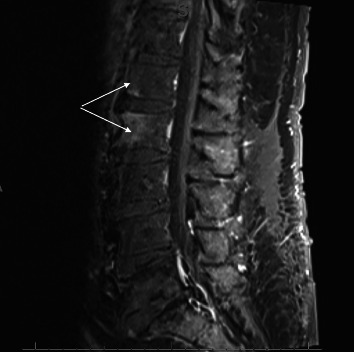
First lumbar MRI (16.12) with white arrow showing signs of L1-L2 spondylitis.
